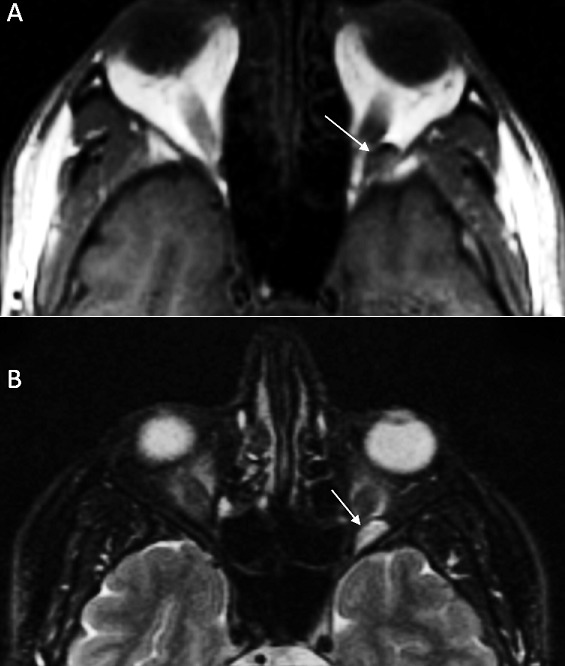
axial images of orbital MRI showing a left intra-orbital process which is low signal in T1 (A) and high signal in T2 (B)
